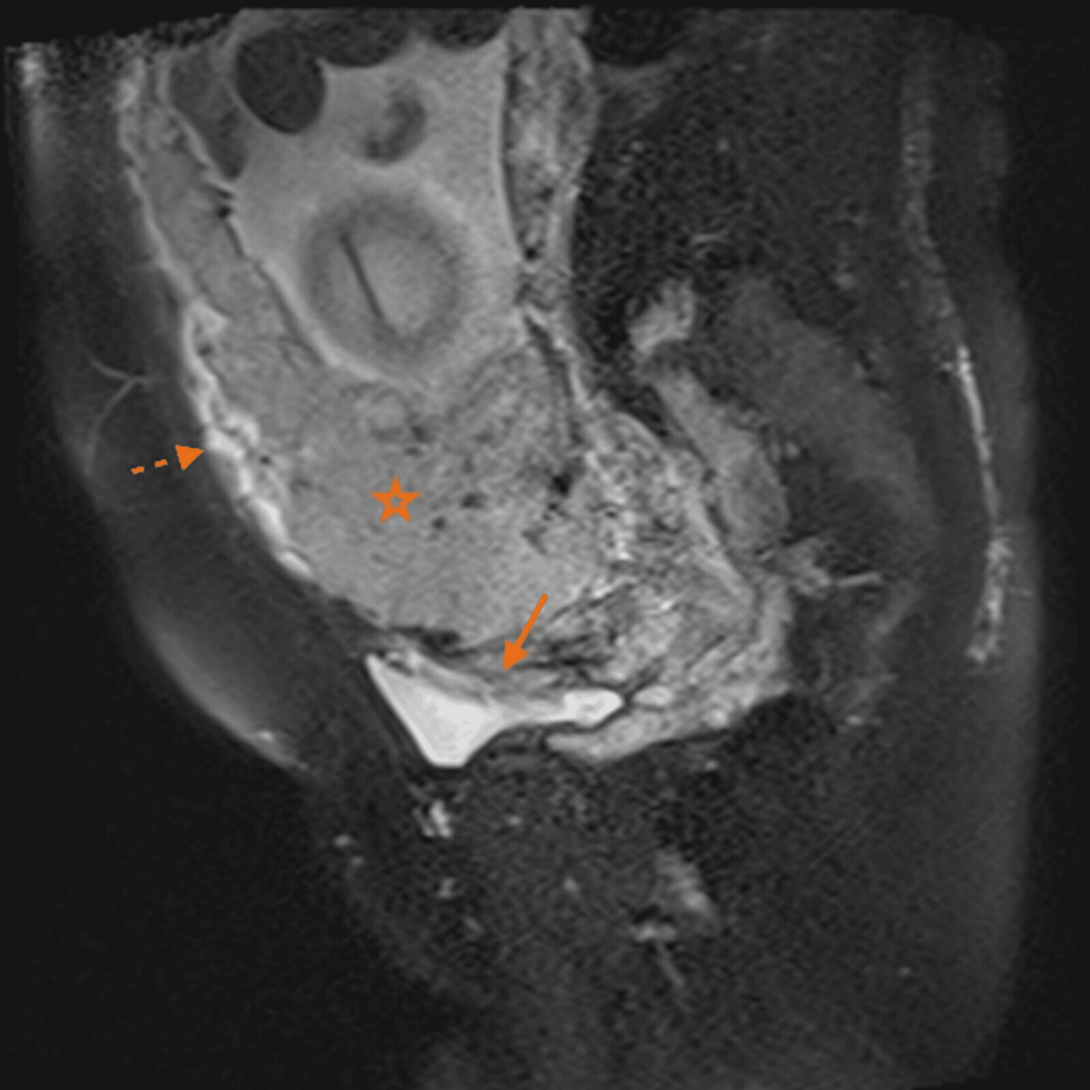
Magnetic resonance imaging in T2 sequence with fat saturation in the sagittal plane. Placenta (star) with irregular morphology, heterogeneous due to the presence of hypointense bands and signal voids. The lower uterine segment is widened. Loss of continuity of the retroplacental hypointense line due to placental invasion (arrow) into the bladder. The myometrium is observed preserved in the anterior uterine segment (dashed arrow).
